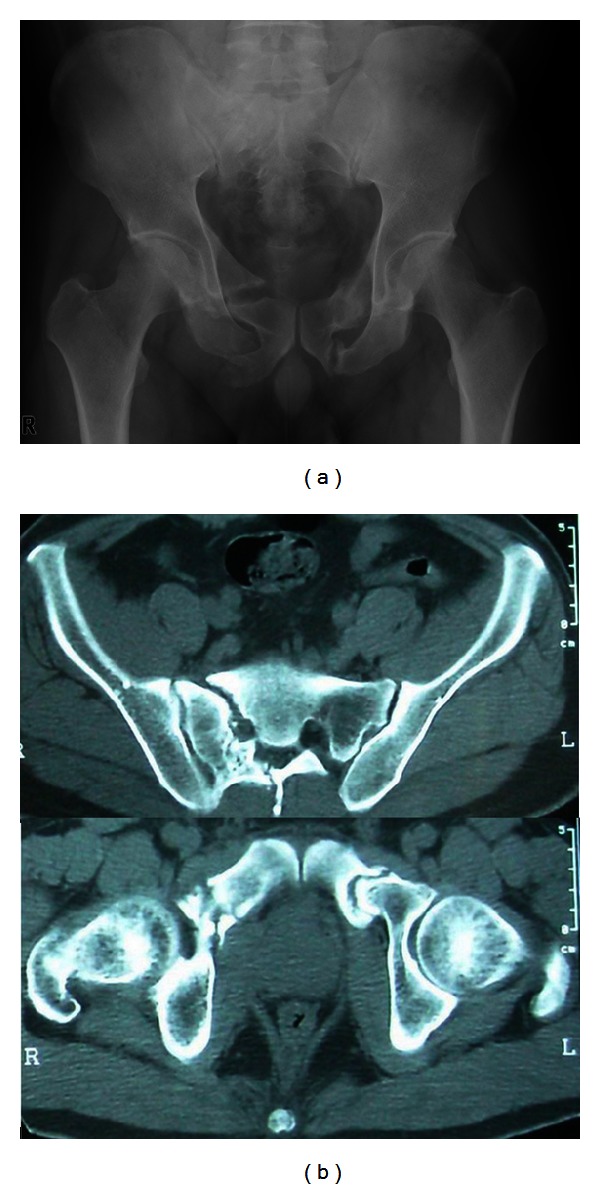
Anteroposterior radiograph (a) and CT images (b) of the pelvis at 5 months after injury, showing nonunion of the right sacrum bone and bilateral pubic rami.
